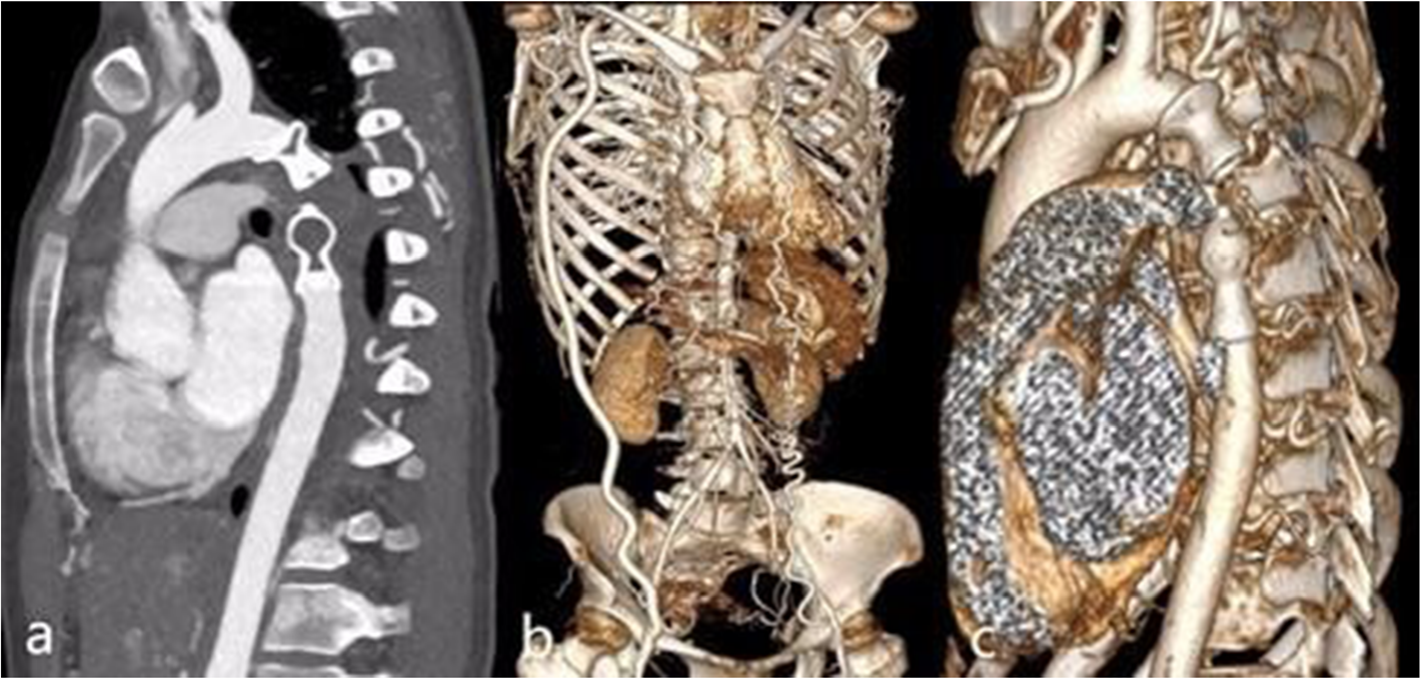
1-year follow-up CT angiography. a. Multiplanar reconstruction imaging (MPR) demonstrated obliteration of the coarcted segment and distal pseudoaneurysm. b. Three-dimensional imaging showed a patent right axillary-to-femoral bypass graft. c. A 3-dimensional image showed the satisfactory position and shape of the occluders
